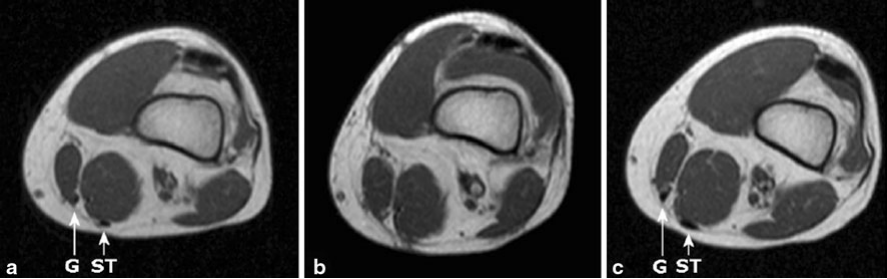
Transverse MRI images of gracilis (G) and semitendinosus (ST) tendons of same patient 6.3 cm proximal to the joint line at time intervals: a preoperative; b 2 weeks postoperatively; c 12 months postoperatively
